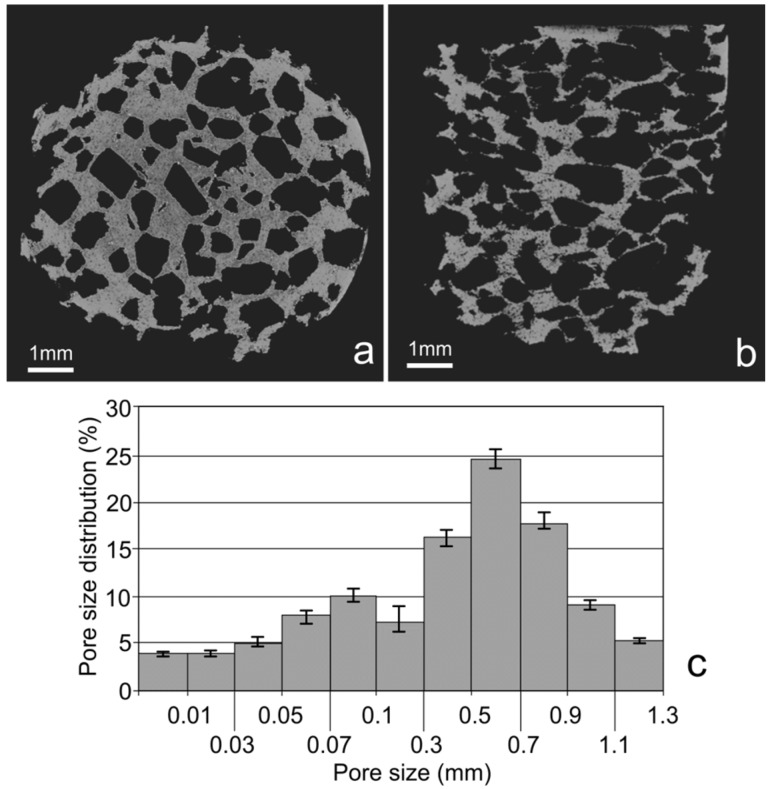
CT cross sections recorded at different sample position—top view (a); top-side view (b); and calculated pore size distribution (c).
